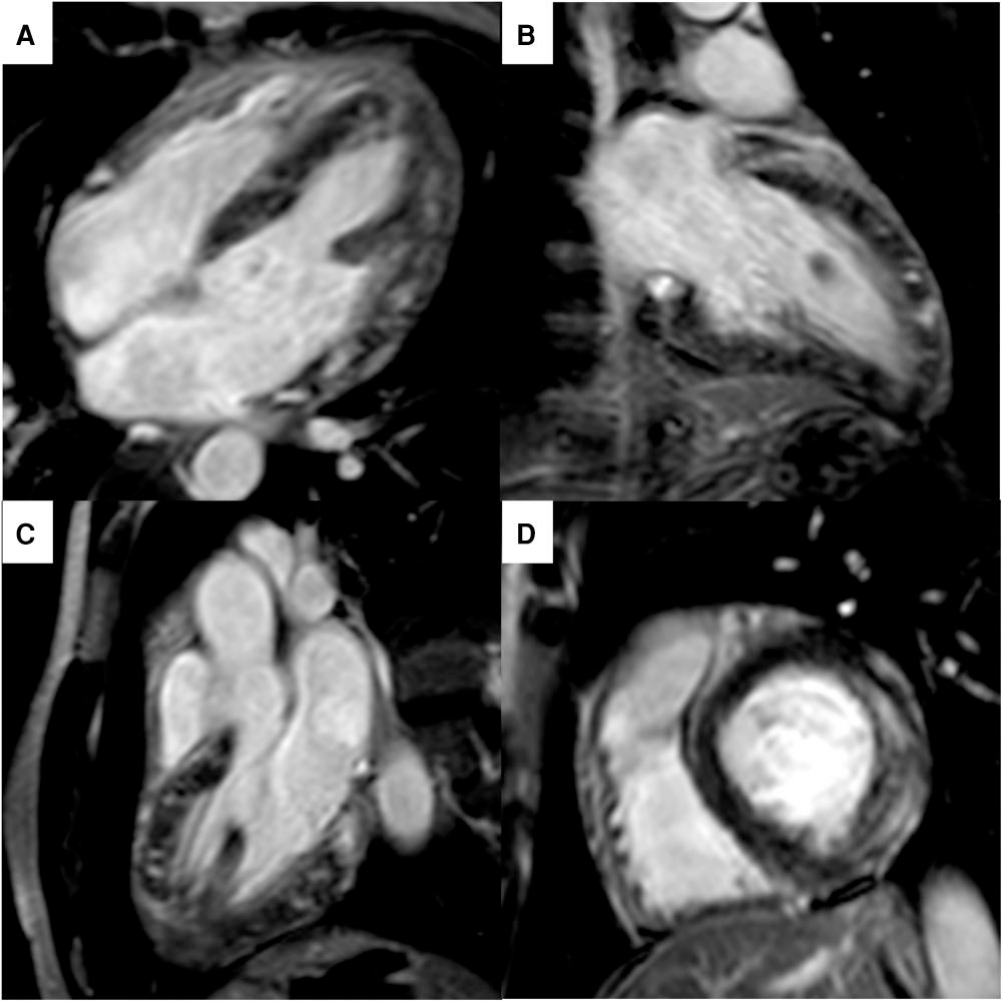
Late gadolinium enhanced cardiac MRI images acquired 3 days following admission. Four-chamber (*A*), three-chamber (*B*), two-chamber (*C*), and basal short axis (*D*) views showing extensive, patchy enhancement in the mid-wall and sub-epicardium. Short axis slice in the basal ventricle (*D*) shows prominent mid-wall and sub-epicardial enhancement in the lateral wall. The lateral wall is visibly thickened compared to the septum (*D*).
